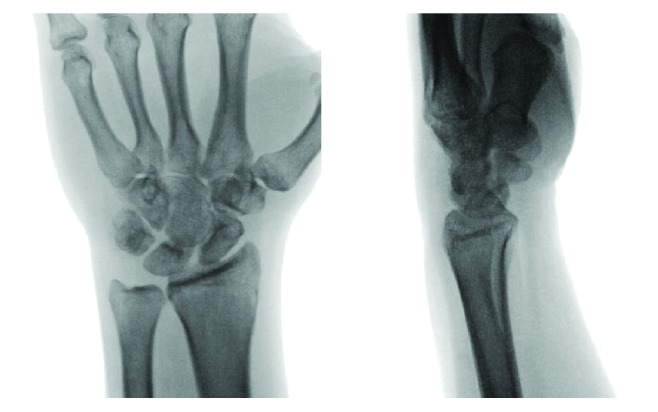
Plain films at the five months postoperative visit.
